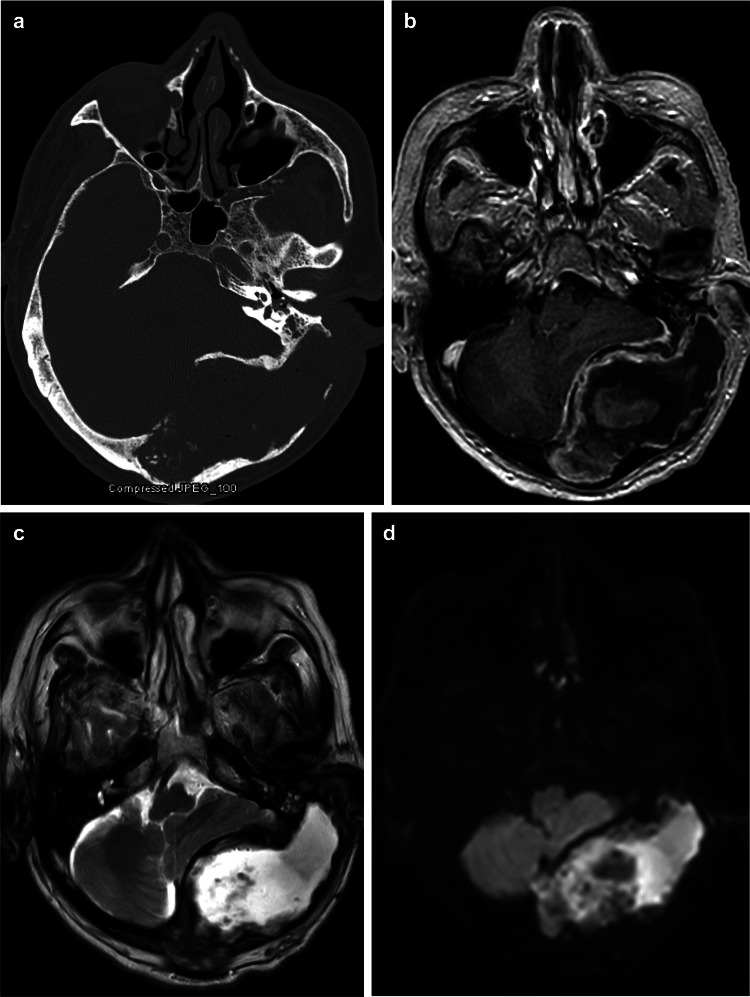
Preoperative imaging. (a) Axial computed tomography showing erosion of the external auditory canal and posterior fossa with an intradiploic lesion in the occiput. Axial magnetic resonance imaging (T1-weighted image) with gadolinium showing rim enhancement (b), T2-weighted image showing an isointensity to cerebral spinal fluid in the lesion (c), and diffusion-weighted image showing bright spots suspicious for an epidermoid tumor with possible infection (d). *Used with permission from Barrow Neurological Institute, Phoenix, Arizona*.
